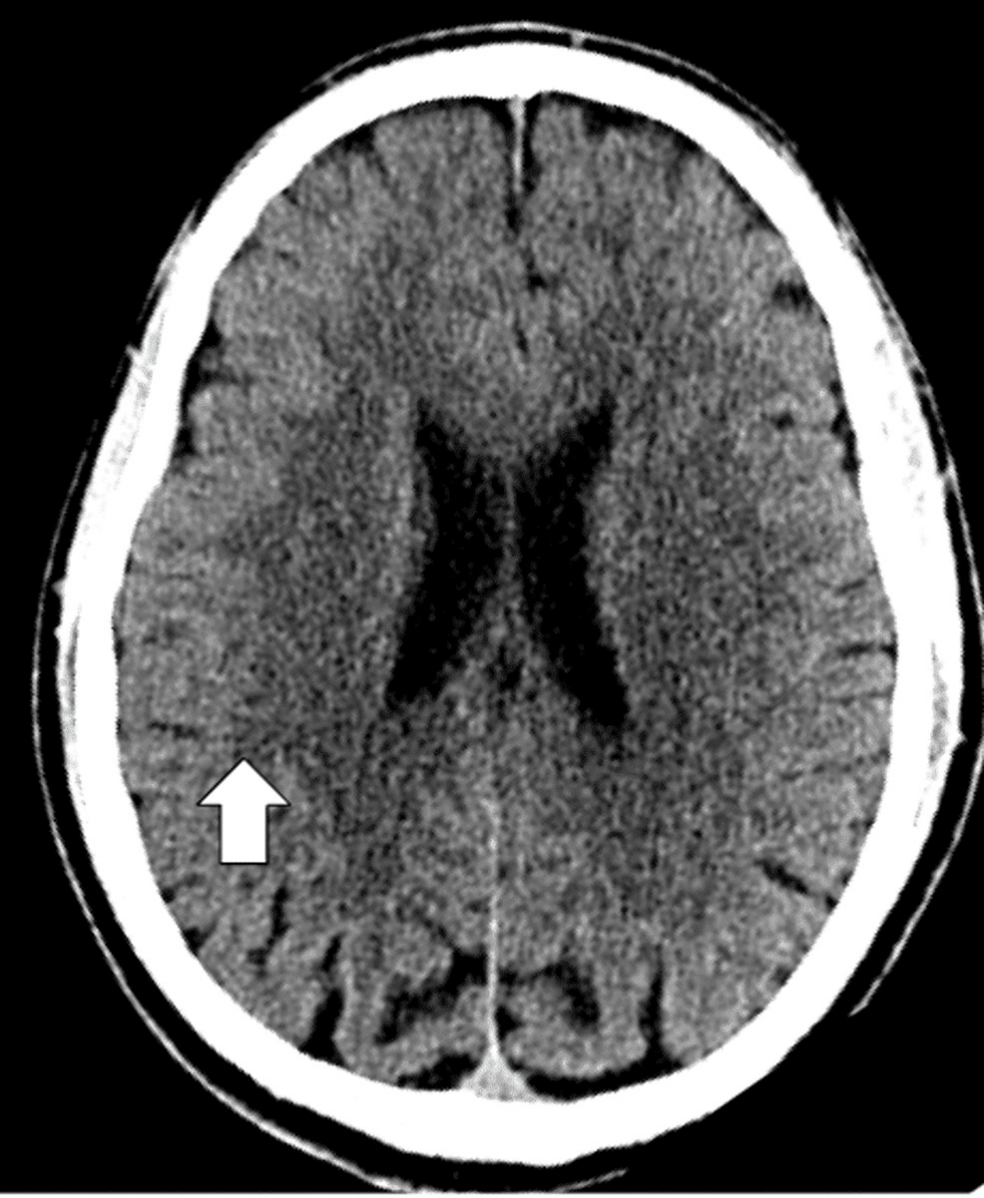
CT head or brain without contrast showing no signs of an acute infarct or hemorrhage with normal grey-white differentiation depicted by white arrow
